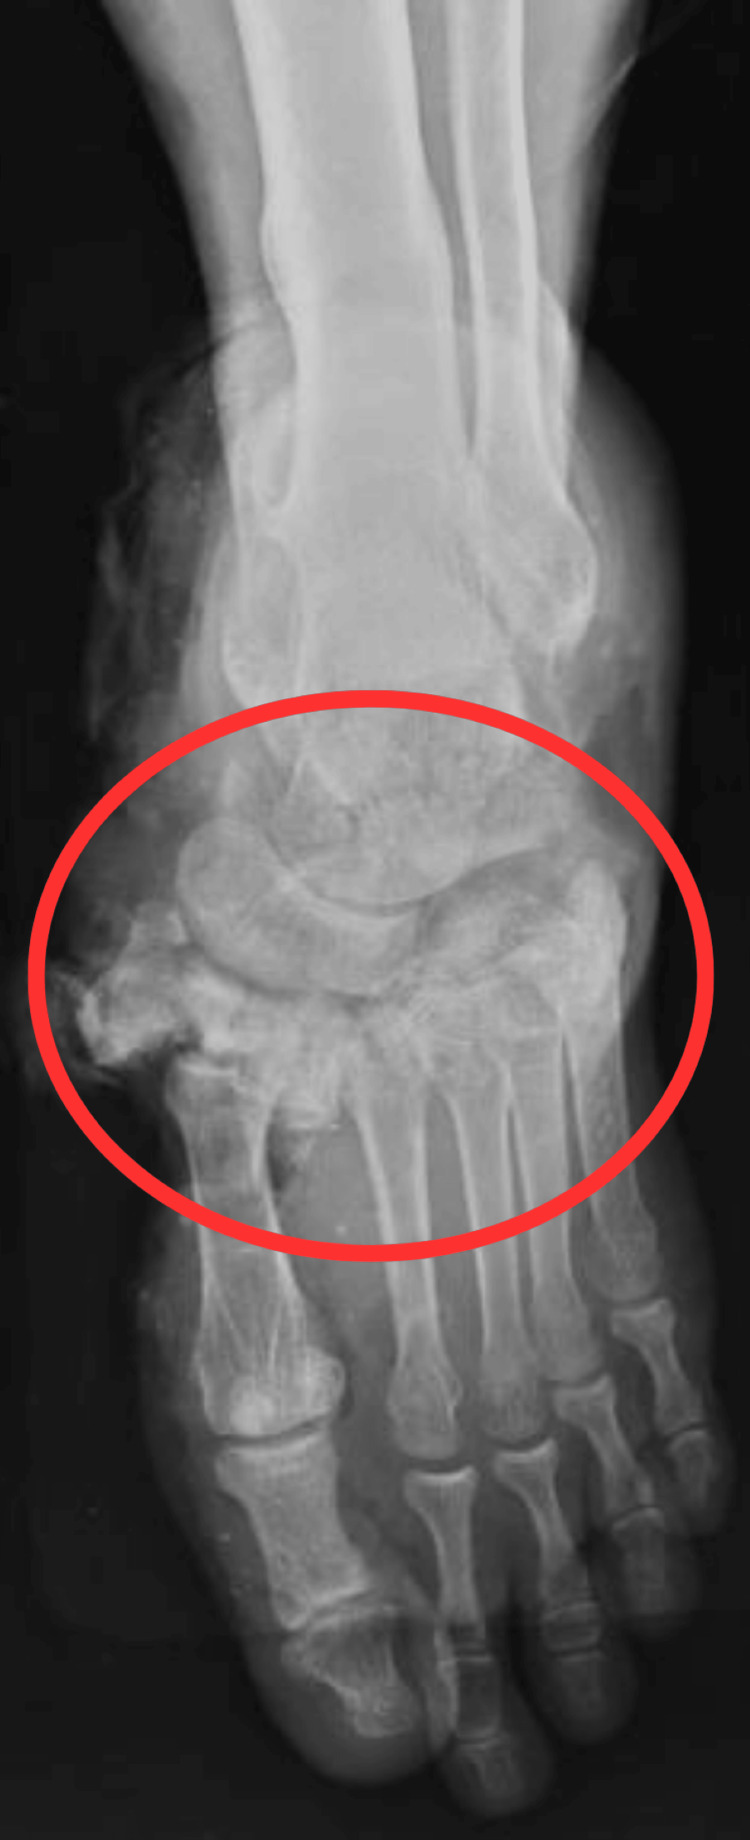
AP view of X-ray of the affected foot The circle shows fractures of the cuneiform and cuboid bone AP, anteroposterior
